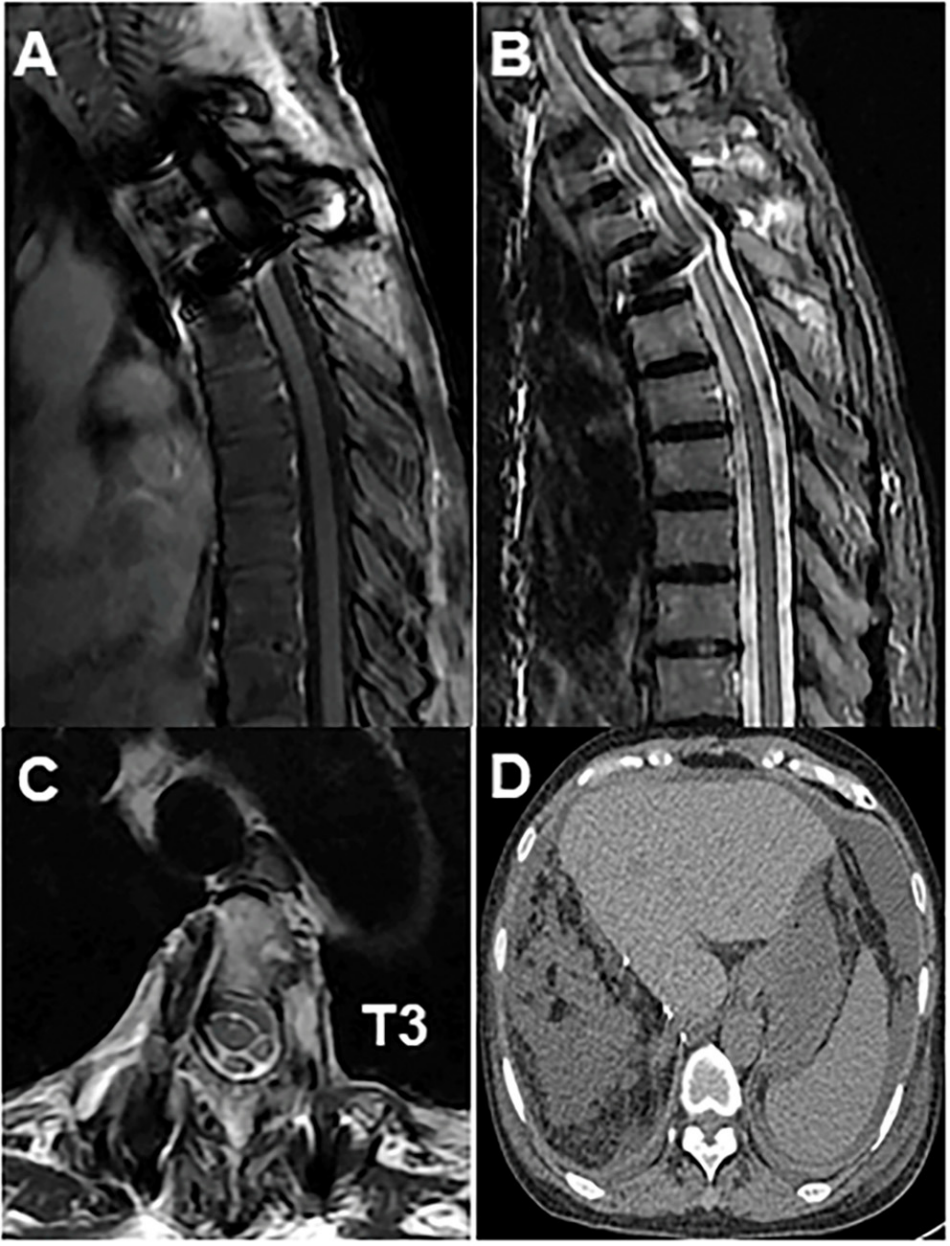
(A) Post-operative radiograph showed fixation of the thoracic spine from T2 to T4; (B) T2-weighted sagittal MRI showed no disease recurrence at T3; (C) axial MRI showed no disease recurrence at T3; (D) abdominal MRI showed no disease recurrence at the liver.
